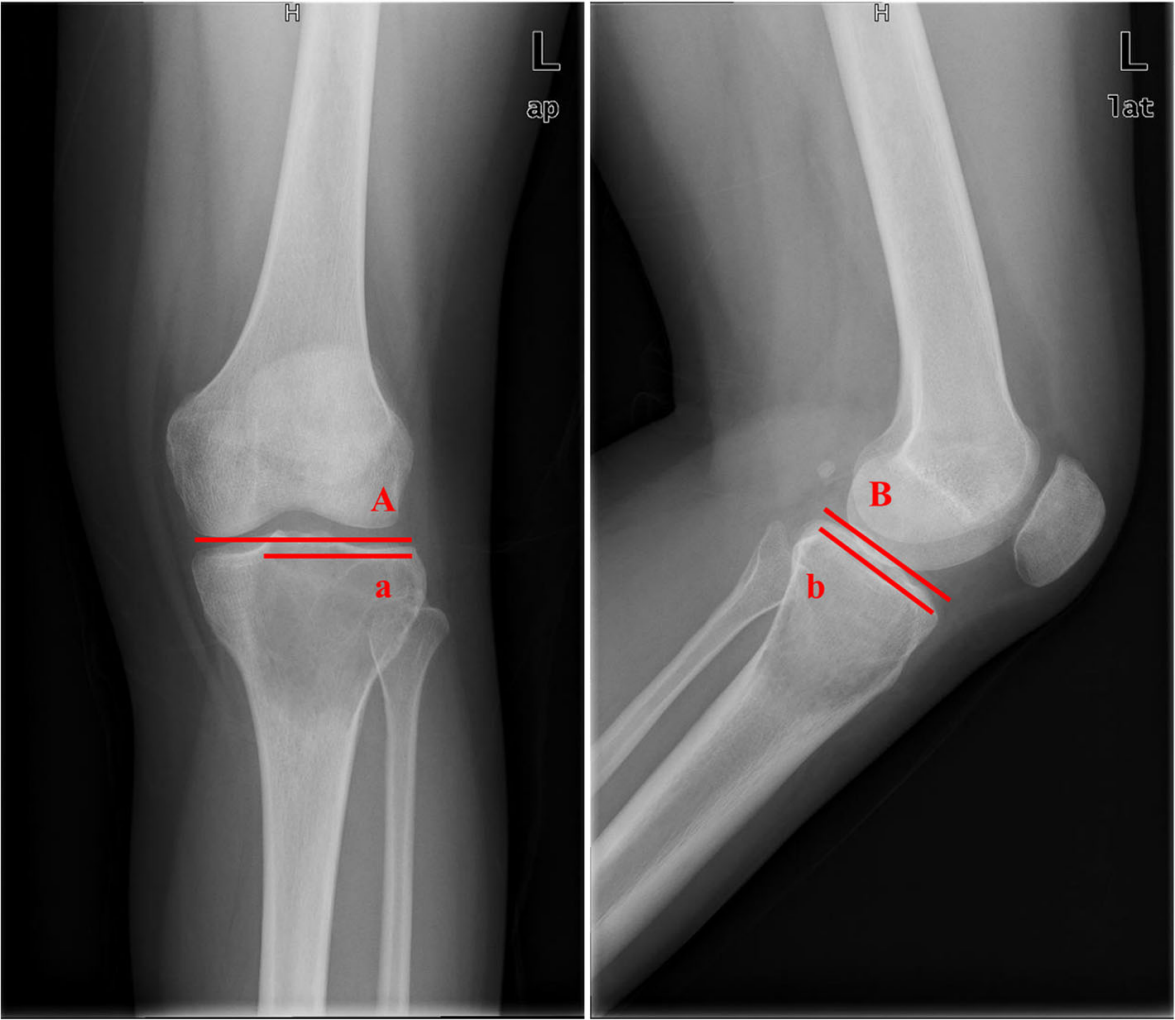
The calculation method of the affected SCB area proportion in the radiograph. A: length of total SCB on anteroposterior view; B: length of total SCB on lateral view; a: length of affected SCB on anteroposterior view; b: length of affected SCB on lateral view
